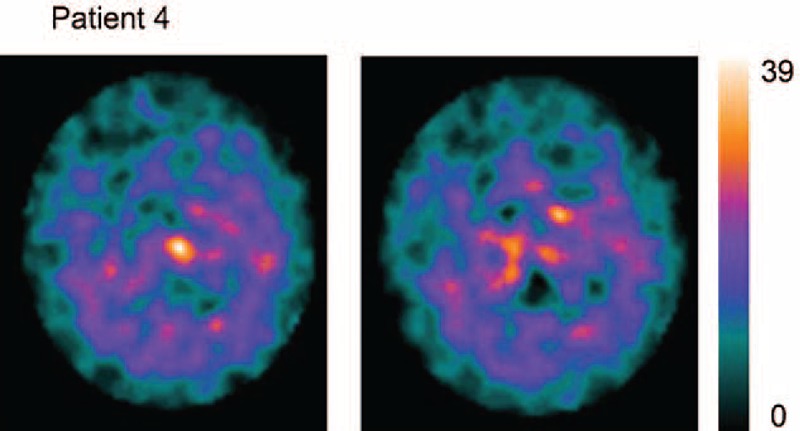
FP/CIT SPECT acquisition in patient 4, who died during AC. SPECT is plotted at the maximum saturation level: notice residual activity in areas corresponding to caudate, and the widespread distribution of the ligand, appearing with low levels in extrastiatal areas. Notice that the saturation level of the scale is 1/4 of scales reported in Figure 3, and a 1/5 to 1/4 of Figure 1. Binding uptake shows the typical pattern “burst striatum,” a severe bilateral reduction with almost no uptake in either the putamen or caudate with increased background uptake.^28,31^. AC = akinetic crisis, SPECT = single-photon emission computerized tomography.
